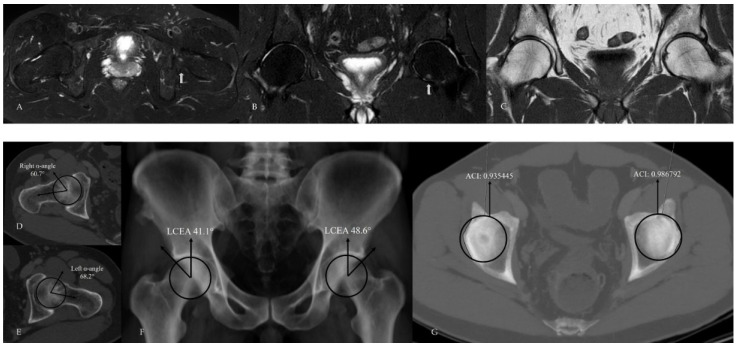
Hip MRI demonstrates focal bone marrow edema in the inferior aspect of the left femoral head on (A) axial and (B) coronal fat-suppressed T2-weighted images (white arrow). (C) Coronal T1-weighted image shows no associated additional imaging findings. (D,E) CT demonstrates alpha angle measurements, and (F) LCEA measurements indicate bilateral mixed-type femoroacetabular impingement. (G) Acetabular overcoverage is confirmed using the ACI.
